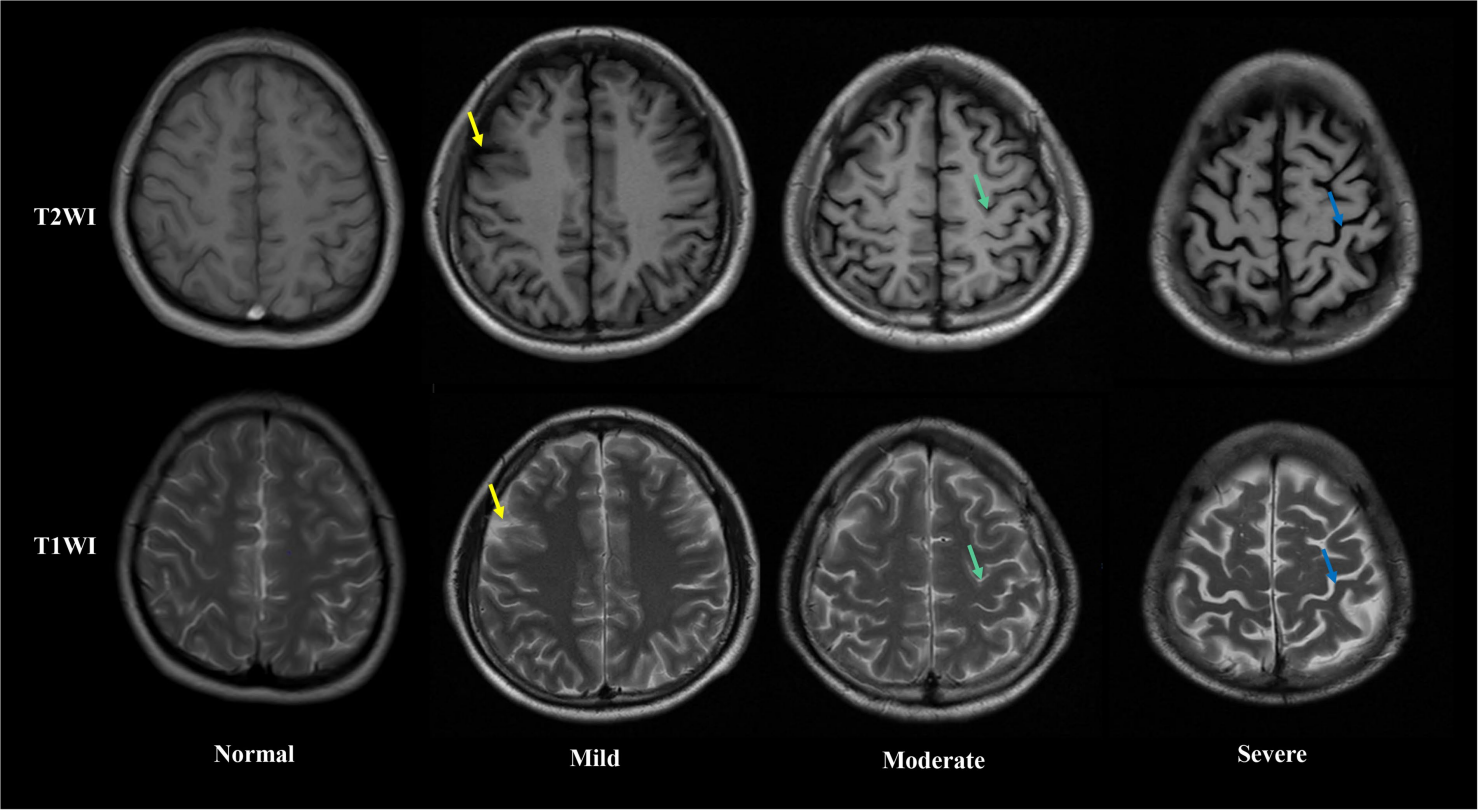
Axial T1 weight (up) and T2 weight (down) magnetic resonance images showing different extent of brain atrophy in Alcohol-dependent patients. From left to right, columns represent absent, low, moderate and sever brain atrophy. In low brain atrophy, sulcal opening peripherally (yellow arrows) is observed. In moderate brain atrophy, widening along the length of the sulcus (green arrows) are observed. In severe brain atrophy, gyral thining (red arrows) is observed.
